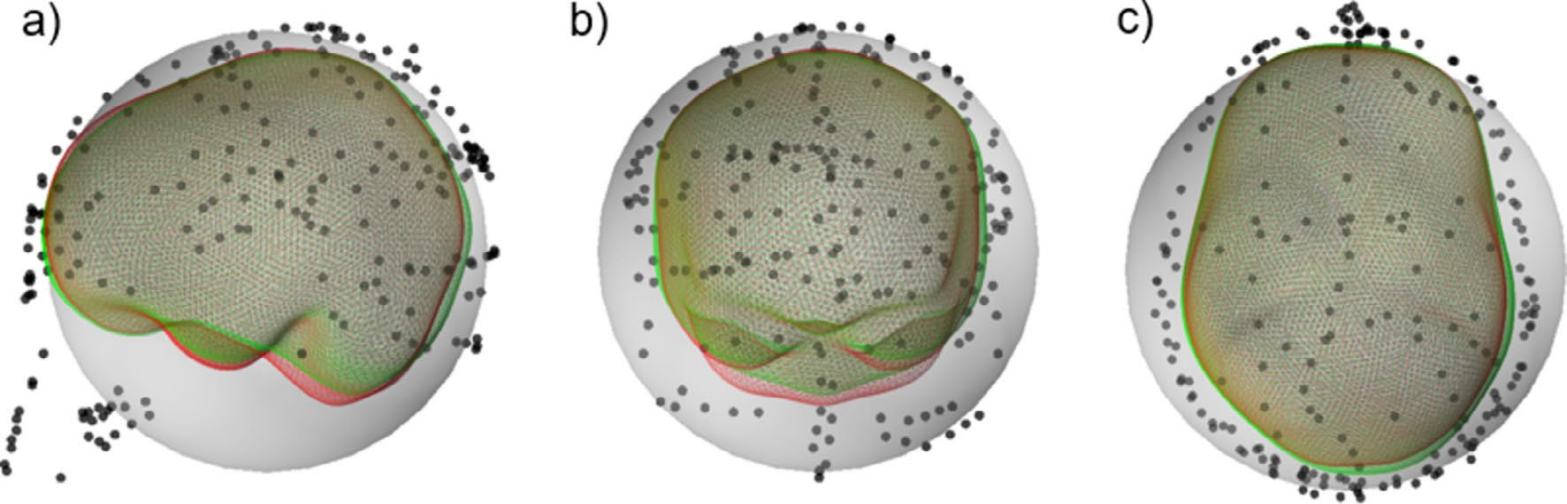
Single‐shell volume‐conductor‐model surfaces from the real‐MRI (green), pseudo‐MRI (red), and sphere (gray) fitted to the scalp digitization points (black): (a) left view, (b) front view, and (c) top view.
